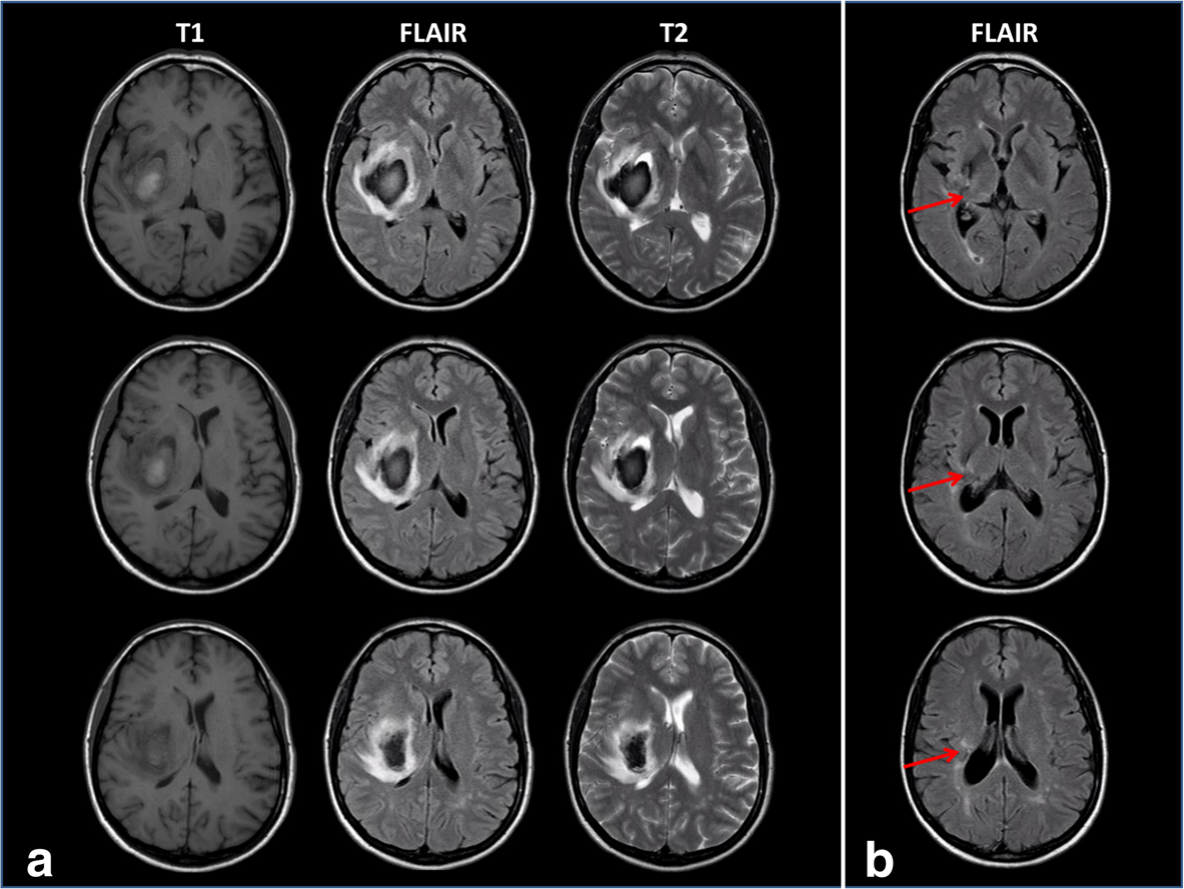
Magnetic resonance images showing the acute right lenticular-capsular-thalamic stroke lesion assessed with T1-weighted, Fluid-attenuated inversion recovery (FLAIR), and T2-weighted sequences (a); one-year follow-up MRI showing the lesion evolution assessed with FLAIR sequence. The red arrows show the posterior thalamic involvement (b)
